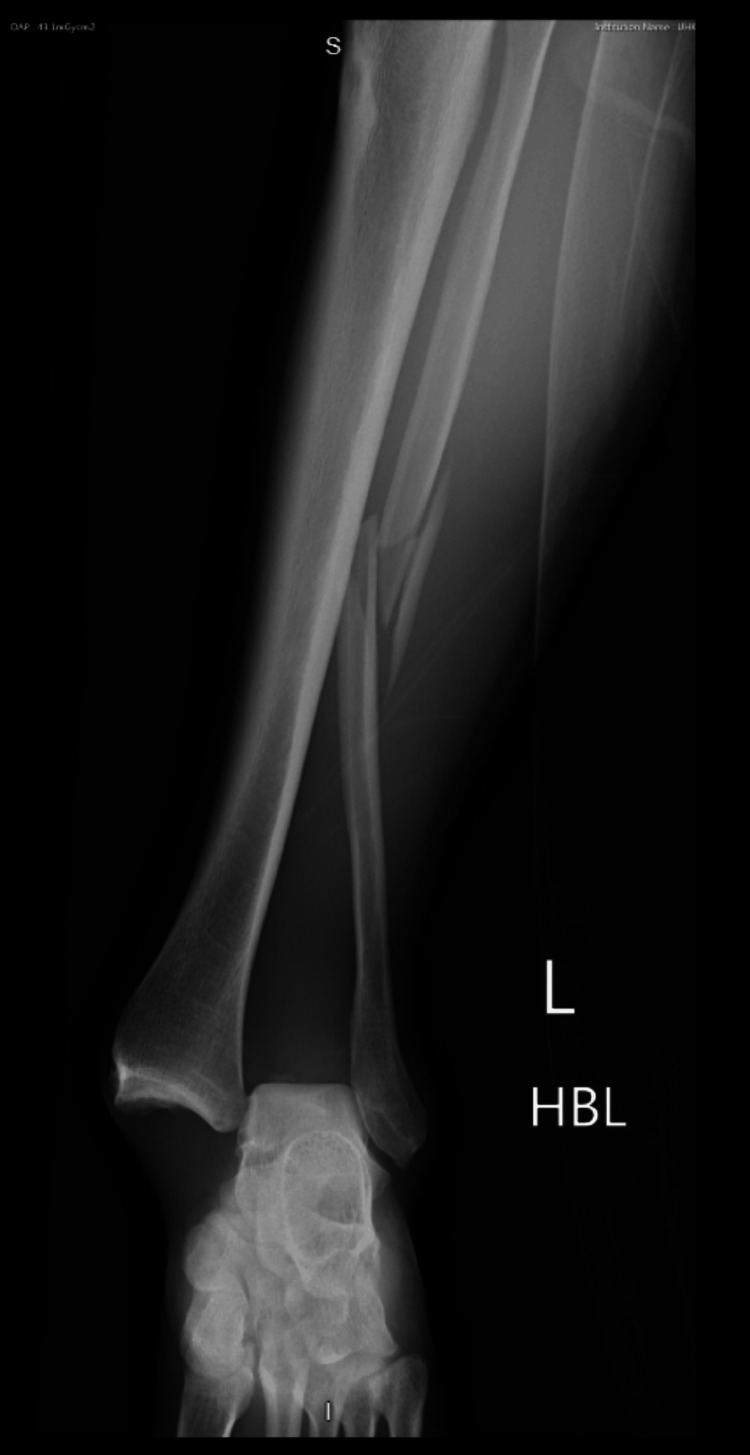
Anteroposterior left ankle radiograph post-tackle playing football
